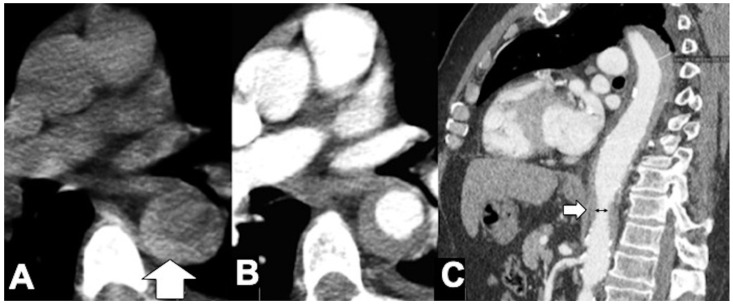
CT features of acute type B IMH in a 62-year-old man with abrupt chest pain and Type B IMH at baseline CTA examination. (A) Axial low-dose unenhanced CT image shows hyperdense crescent sign of the aortic wall (arrow). (B) Axial arterial phase CTA image in the same patient shows decreased diameter of the aortic lumen and the smooth luminal-wall interface. (C) Sagittal reconstruction CTA image shows decreased diameter of the aortic lumen (arrow) and a relative constant circumferential relationship with the wall.
